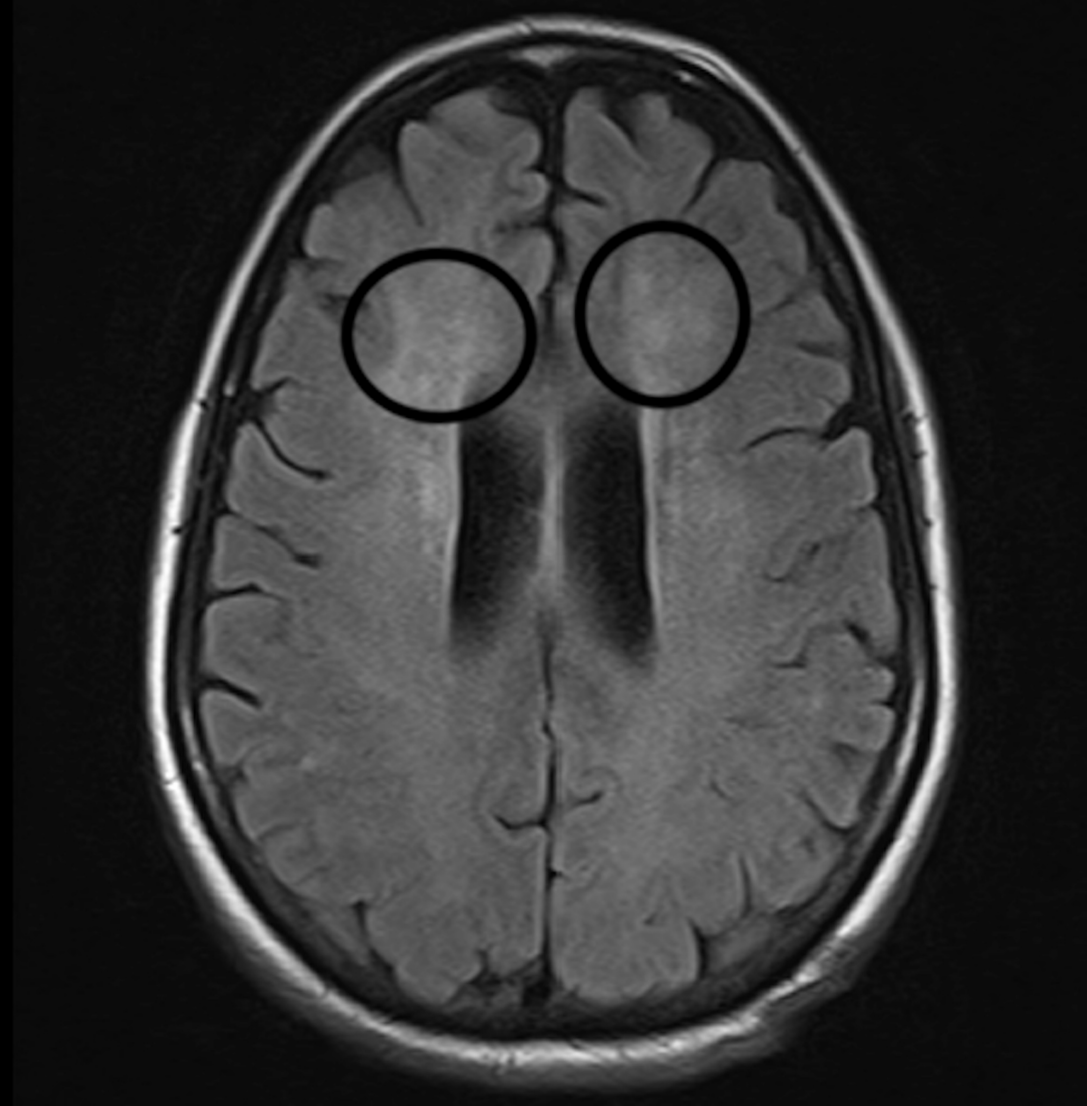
Magnetic resonance imaging (MRI). MRI brain showing nonspecific white matter changes supratentorially (circles).
